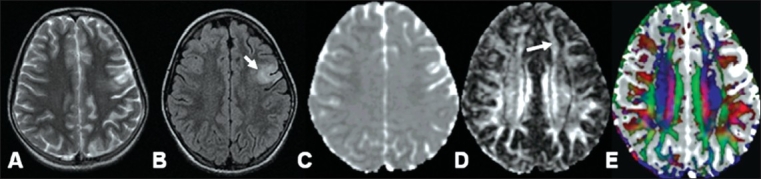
T2W (A), FLAIR (B), and MD map (C) images show an infarct in the left frontoparietal region (arrow) in a 30-year-old woman. The corresponding FA map (D) and the color-coded FA fused with MD map (E) images show low FA in the infarcted region (arrow) as well as in the area subjacent to the infarct, which appears normal on conventional imaging 6 weeks after the onset of stroke
